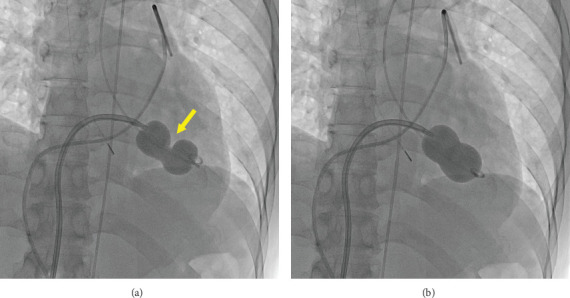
X-ray fluoroscopy during quick inflation of the Inoue balloon demonstrating a deep indentation of the balloon (a, *arrow*) shortly followed by full extension of the balloon (b).
